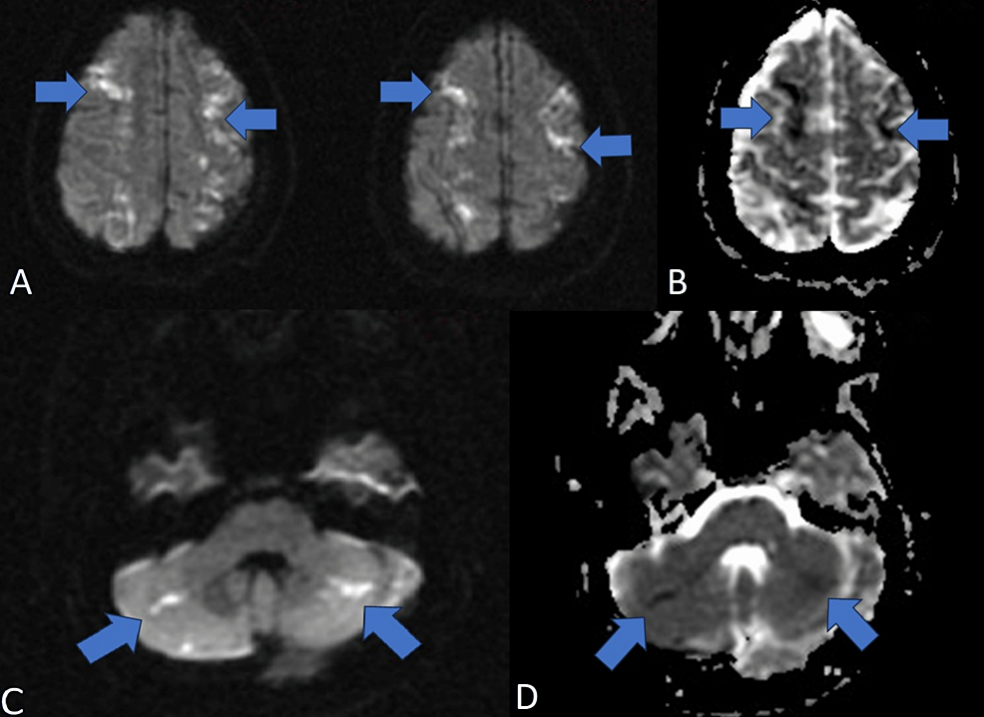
MRI head showing multiple areas of stroke suggesting thromboembolic showering (A) DWI images showcasing areas of bilateral frontal lobe infarcts (blue arrows). (B) ADC image showing areas of bilateral frontal lobe infarcts (blue arrows). (C) DWI image showing areas of bilateral cerebellar infarcts (blue arrows). (D) ADC image showing areas of bilateral cerebellar infarcts (blue arrows). DWI: diffusion-weighted imaging; ADC: apparent diffusion coefficient
